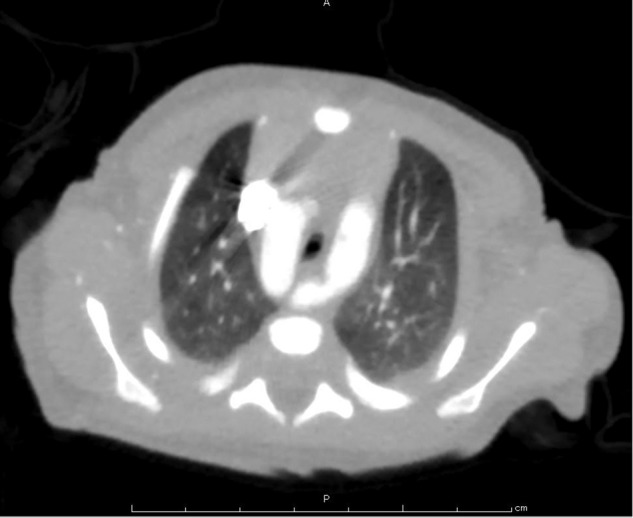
A contrast computed tomography scan on the 3rd day of life. The right aortic arch, aberrant origin of the left subclavian artery, and left arterial duct formed a vascular ring.
